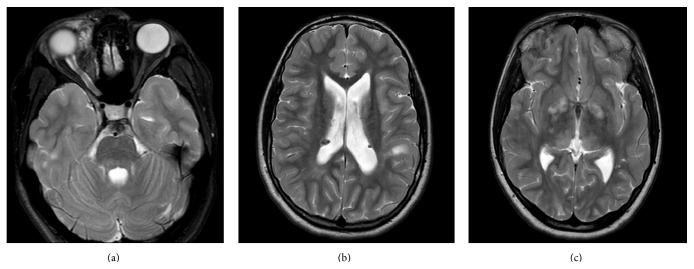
MRI images of the patient. Axial T2 images demonstrating (a) a right anteromedial orbital plexiform neurofibroma, (b) subependymal nodules and a cortical tuber, and (c) hyperintense signal in the thalamus and globus pallidus.
